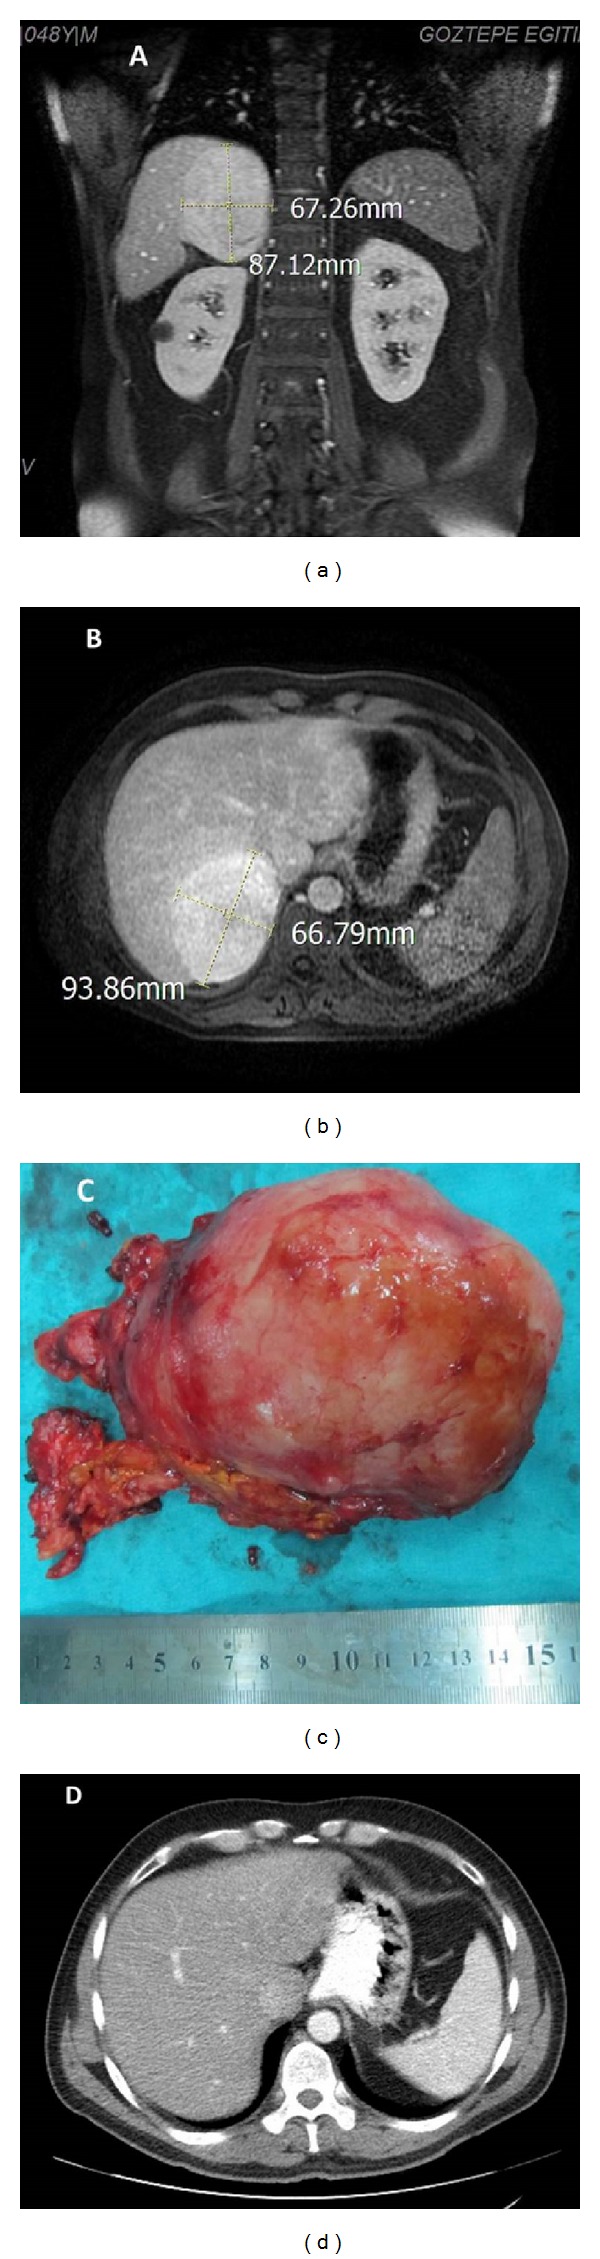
Magnetic resonance images of a 9 cm right adrenal leiomyosarcoma. T1-weighted images in coronal section (a) and transverse section show a homogenous solid mass in the right suprarenal area. (c) Macroscopic appearance of roundish, fibrous neoplasia. (d) Six months after right adrenalectomy, postoperative abdominal computerized tomography demonstrated no signs of local recurrence.
